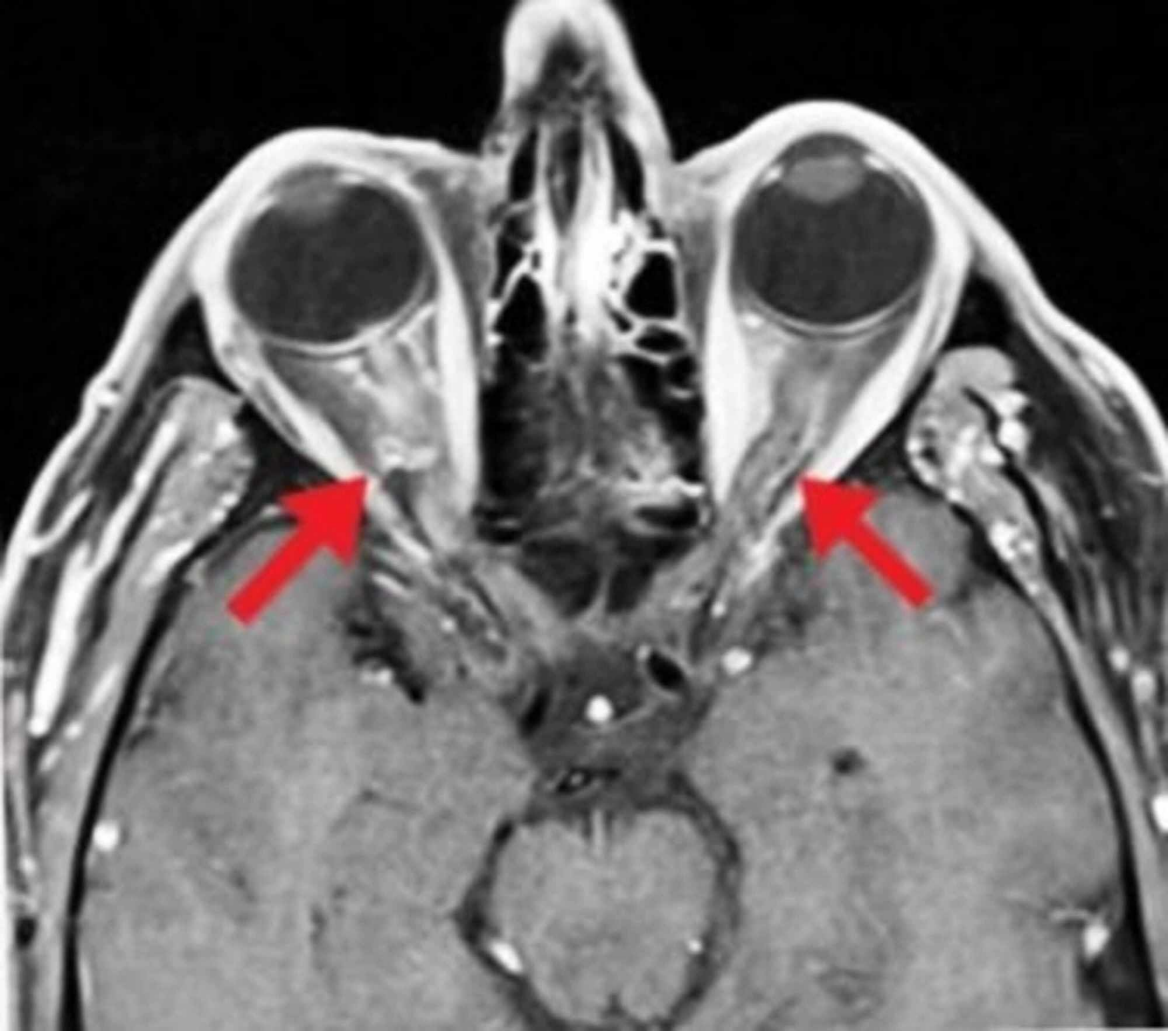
Magnetic resonance imaging of the brain showing bilateral optic nerve thickening
